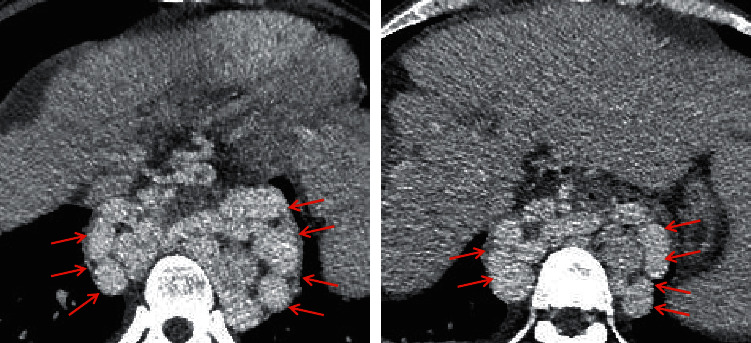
Paraesophageal varices. In a 37-year-old male with liver cirrhosis, the axial contrast-enhanced CT scan on the portal vein phase demonstrated that paraesophageal varices (red arrow) were tortuous vessels outside the esophageal wall.
